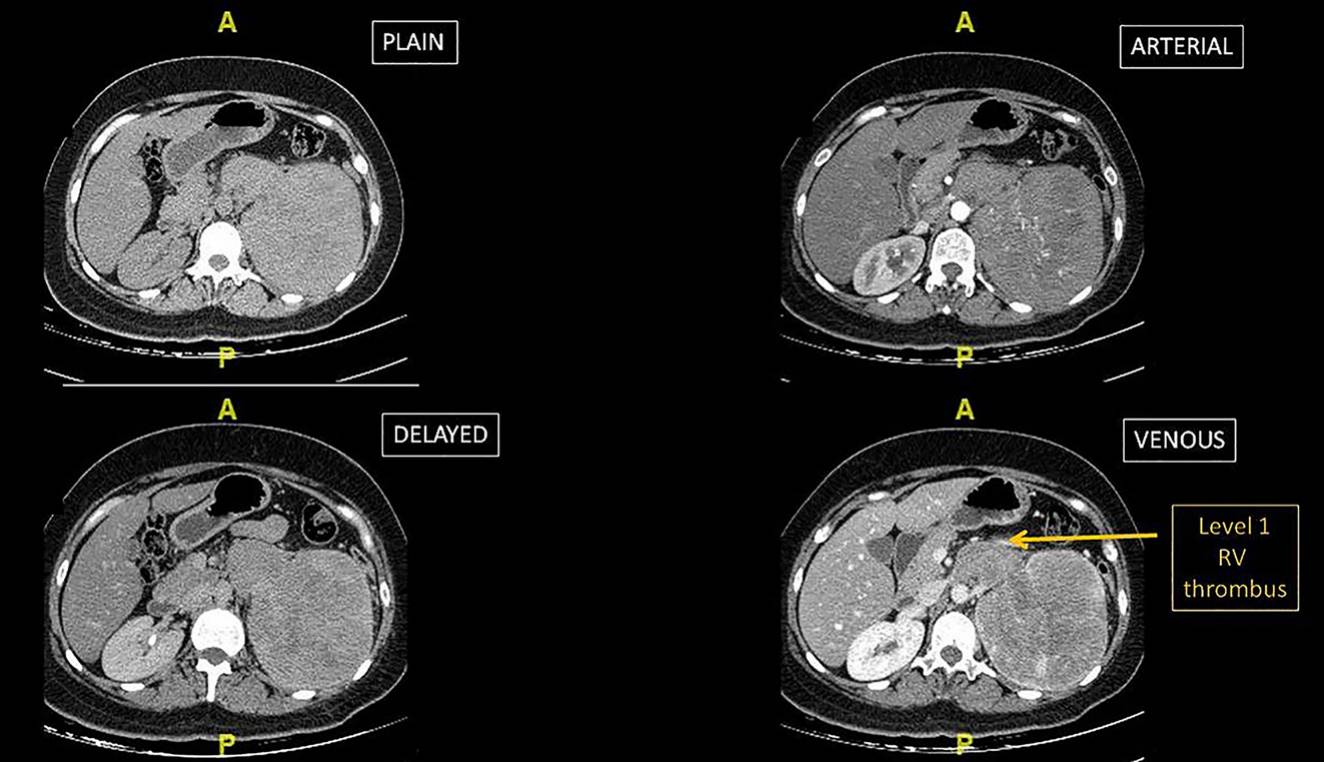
CT scan abdomen- different phases, showing the Level 1 renal vein thrombus.
